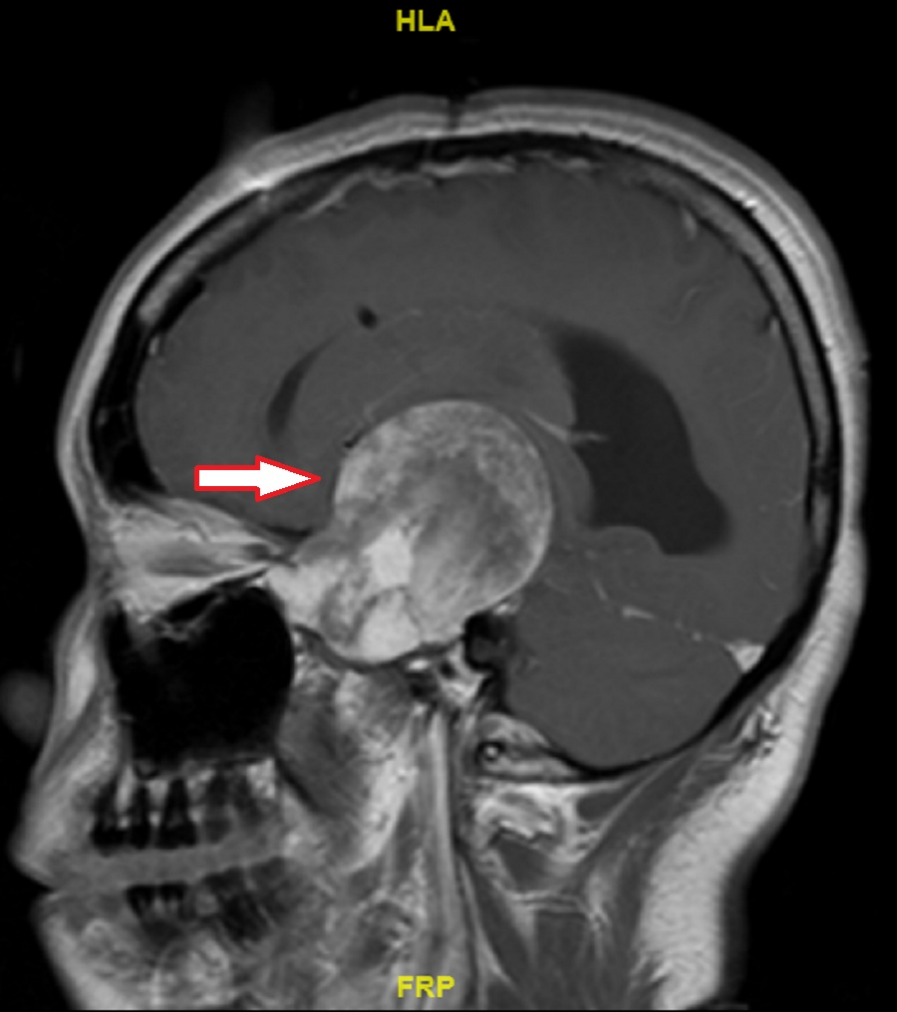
Initial T1 Contrast-enhanced Magnetic Resonance Imaging Sagittal view of tumour (arrow)
